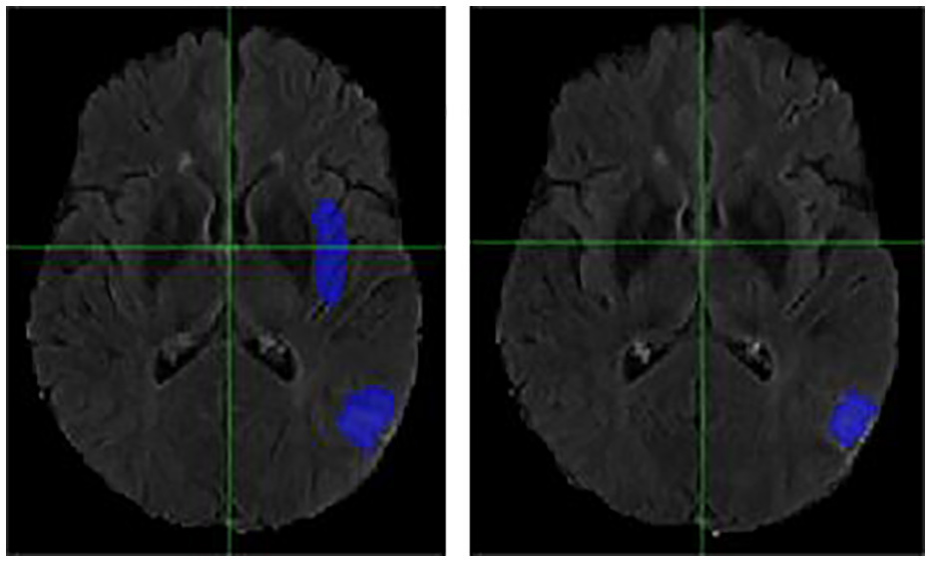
Case One (Improved). Flair MRI. rMR only (Left) and Total SynMR MRI (Right) DSC of WT was improved from 0.21 to 0.67.
